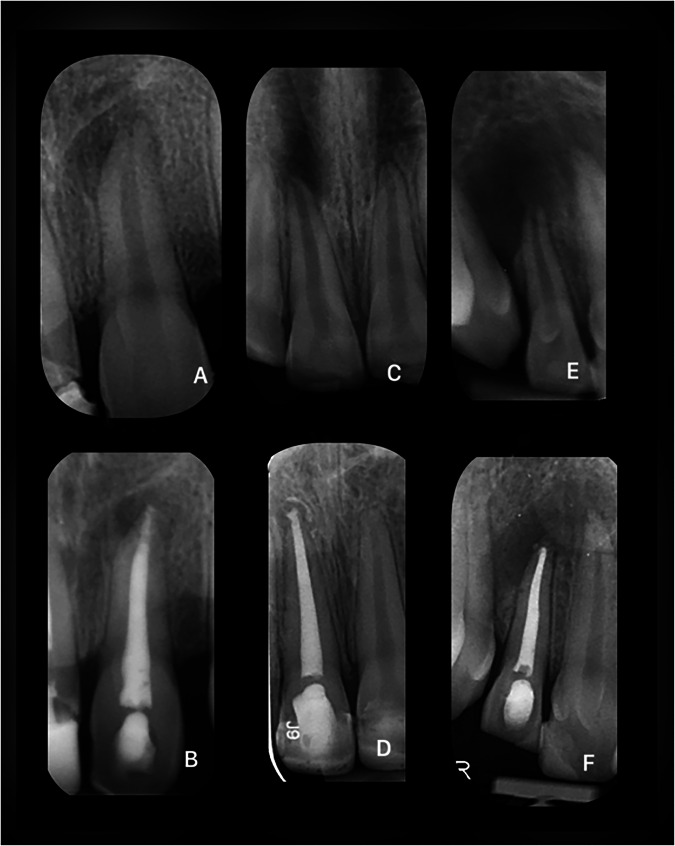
Pre- and post-operative radiographs of example cases with an “Uncertain” outcome. A Maxillary central incisor in an adolescent patient with a history of unknown trauma (Pre-op). B The same tooth at 4 months post-treatment. C Maxillary central incisor in a patient between 5 and 10 years old with a history of enamel-dentine fracture (Pre-op). D The same tooth at 11 months post-treatment. E Maxillary lateral incisor in an adolescent patient with a history of unknown trauma (Pre-op). F The same tooth at 15 months post-treatment.
